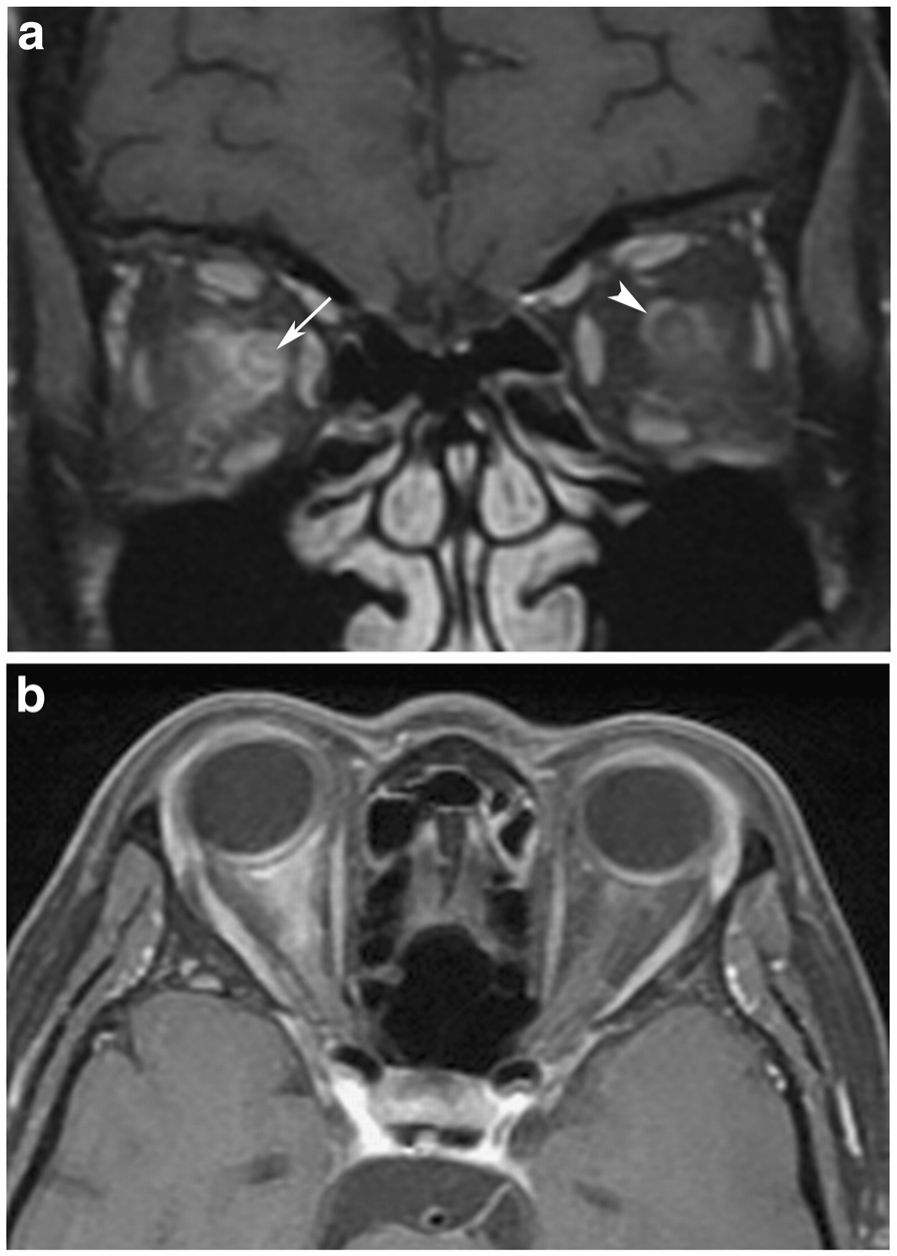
Magnetic resonance imaging. (a) Coronal post-gadolinium fat-saturated T1-weighted image shows enhancement and stranding in the intraconal fat bilaterally, worse on the right. The right optic nerve enhances (arrow head) along with enhancement of the optic sheath. Left perineural enhancement posterior to the globe is also present, as indicated by the arrow. (b)Axial post-gadolinium fat-saturated T1-weighted image demonstrated the marked enhancement in the right intraconal fat.
